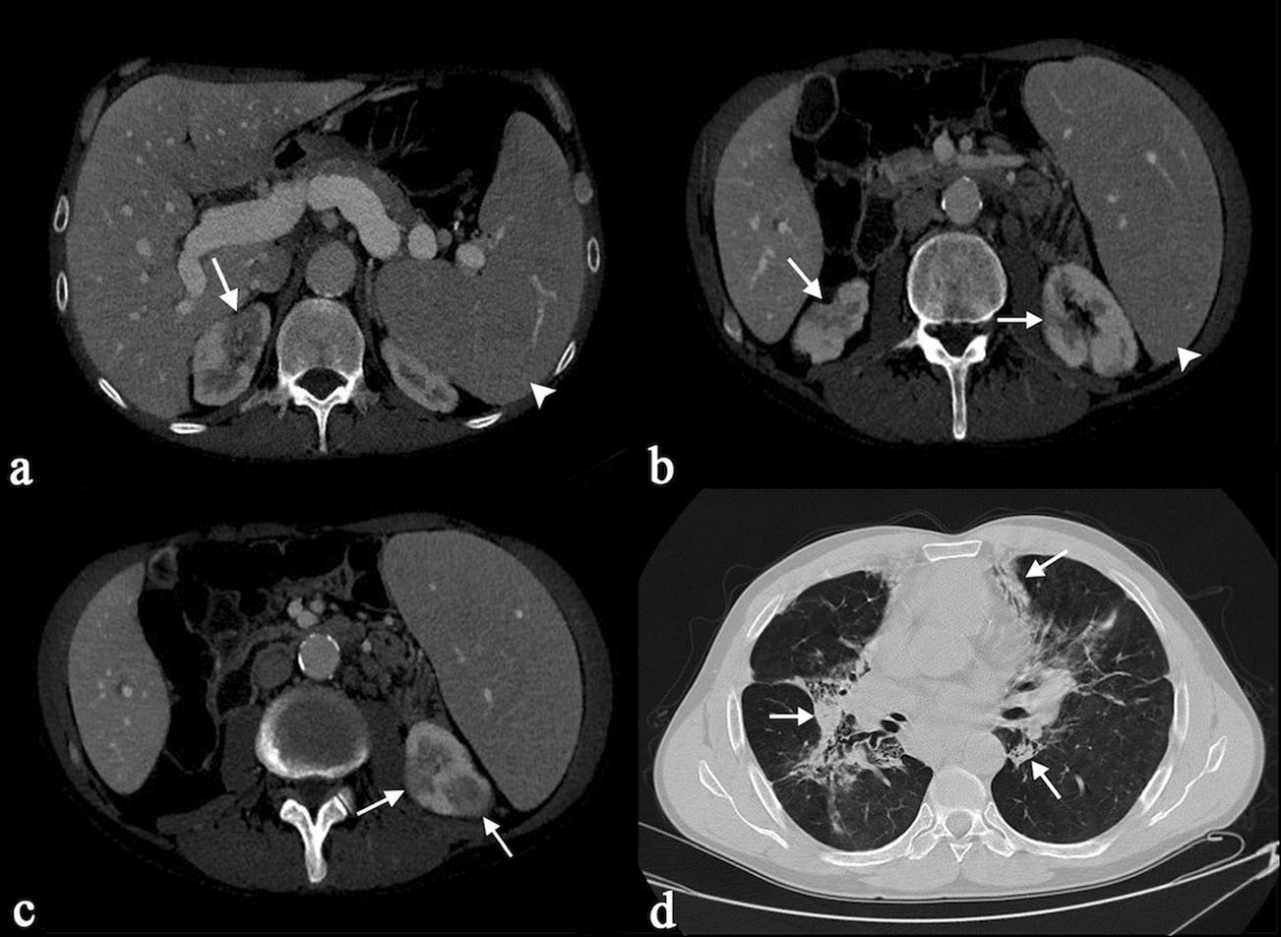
Multiorgan sarcoidosis, with multiple hypodense lesions in the renal parenchyma (*white arrows* in Fig. 12a–c). In the left kidney, in the lower part of the parenchyma, lesions have nodular appearance. Splenomegaly can also be observed (*white arrowheads*). In the Fig. 12d, a sarcoidosis pattern is observed on HRCT images (*white arrows*). Sarcoid lesions in the renal parenchyma were confirmed by biopsy
